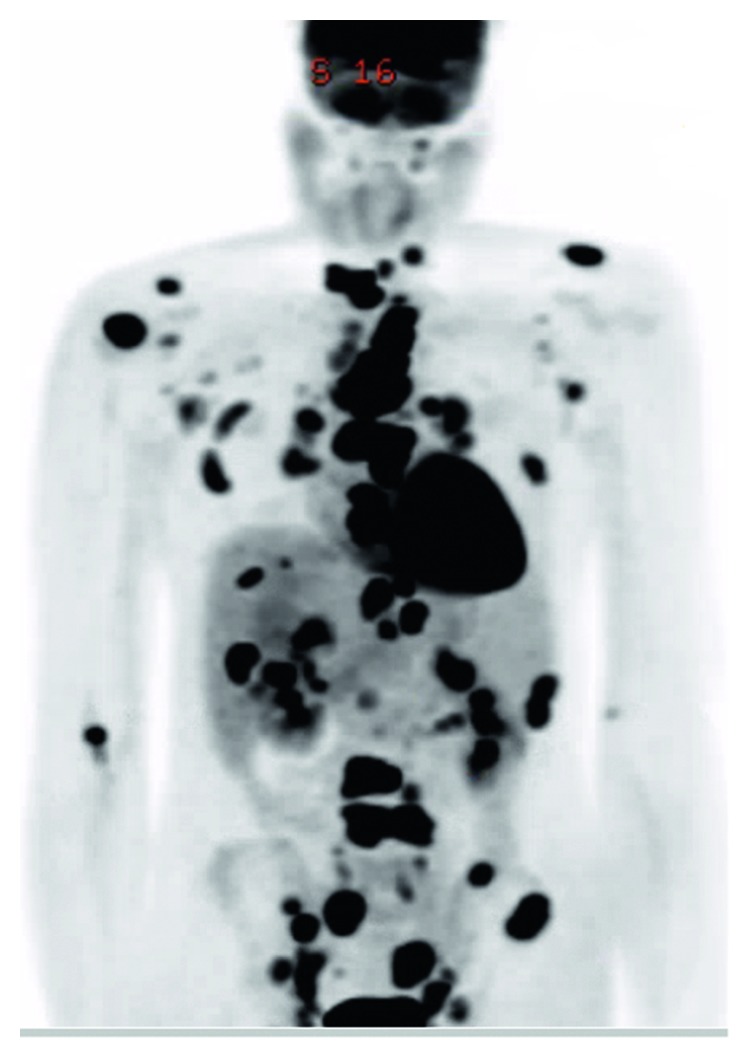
FDG-PET scan extensive hypermetabolic osseous and nodal disease.
